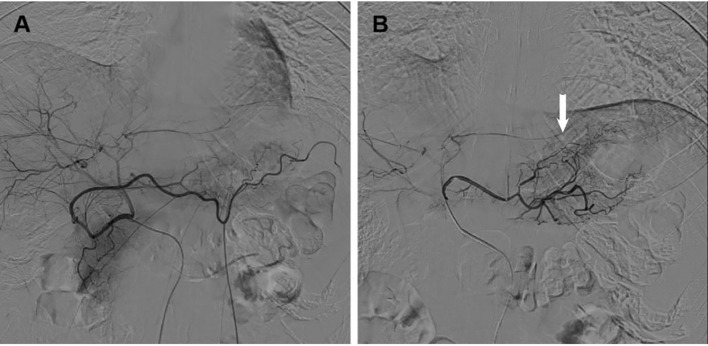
X-ray images of catheter retention positions (A) Angiography of the RH catheter in the celiac axis; (B) Angiography of microcatheter in tumor blood supplying arteries (Arrow: Tumor blood supplying arteries).
